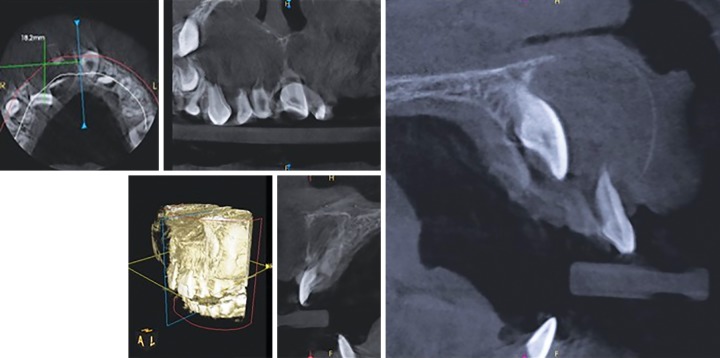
Cone beam computed tomography showing radicular cyst of 51, with apical displacement of 11
